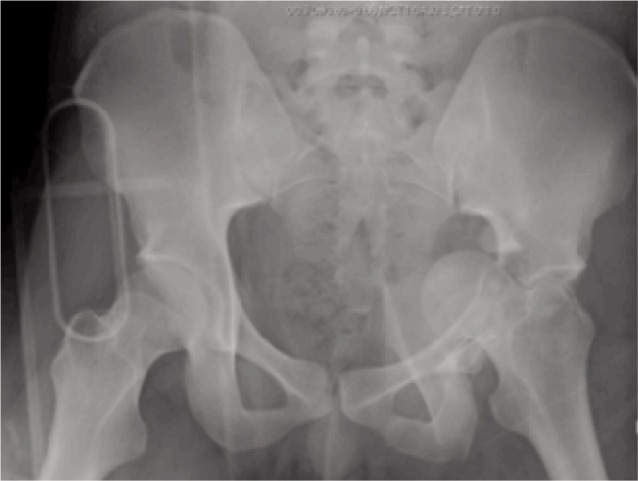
Radiograph of Case One showing T-Type Acetabular fracture.
